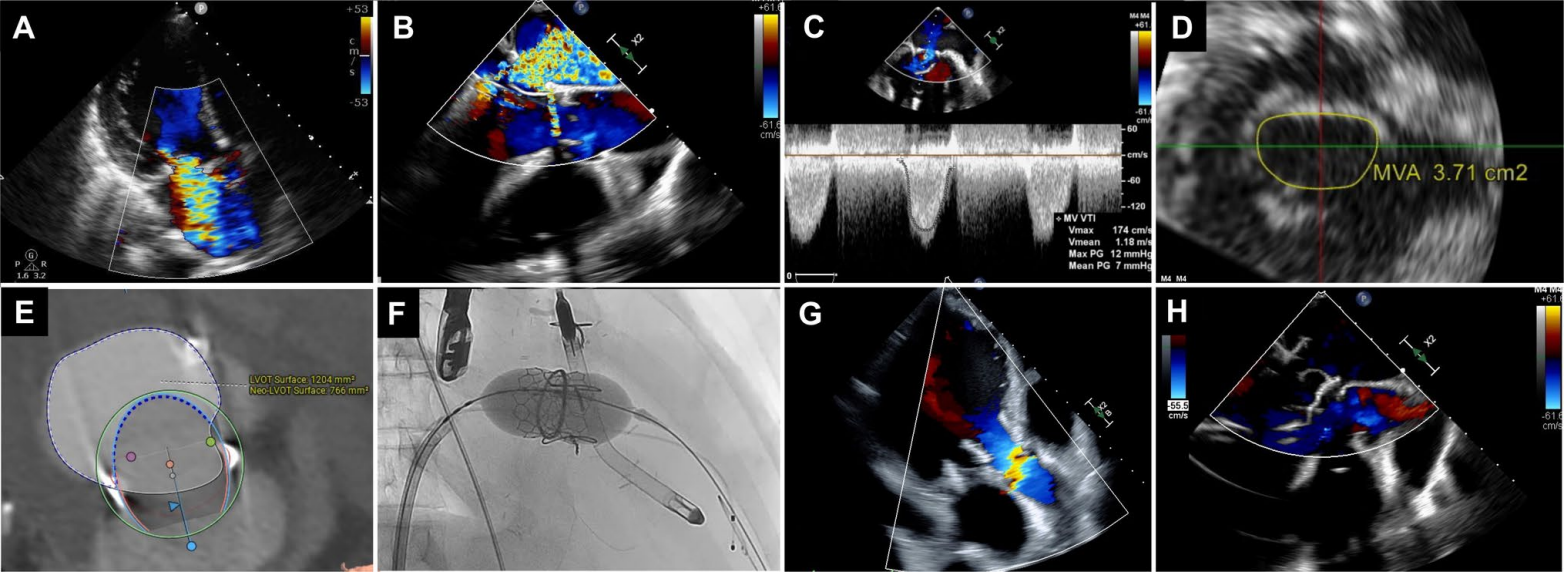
A Preoperative transthoracic echocardiography (TTE), B–D preoperative transesophageal echocardiography (TEE), E Preoperative CT demonstrated a neo-left ventricular outflow tract area measuring 7.66 cm^2^, F transcatheter mitral valve replacement in a failed ring, G postoperative TTE, and H postoperative TEE
